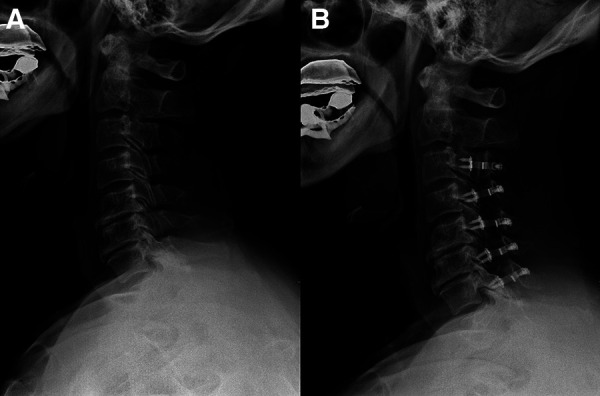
A 65-year-old male patient treated with C3–C7 laminoplasty. (A) Preoperative lateral cervical radiograph (CSVA = 15.4 mm, T1S = 23.4°). (B) Lateral cervical radiograph at final follow-up (17 months after surgery, CSVA = 17.5 mm).
